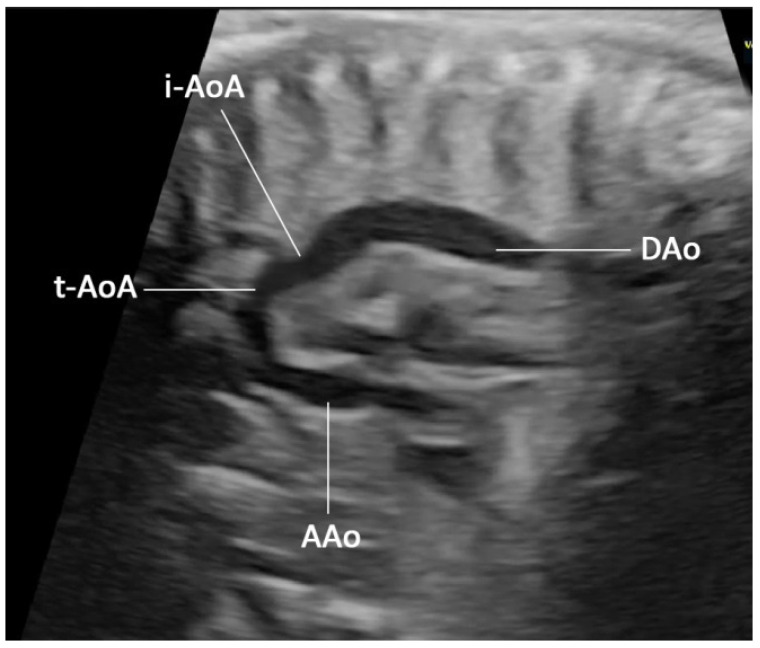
Prenatal ultrasound, sagittal scan, or long-axis view of the aorta shows a small aorta arch (tubular hypoplasia) connecting to the descending aorta with shelf appearance. (AAo: ascending aorta; i-AoA: isthmic aortic arch; t-AoA: transverse aortic arch; DAo: descending aorta).
